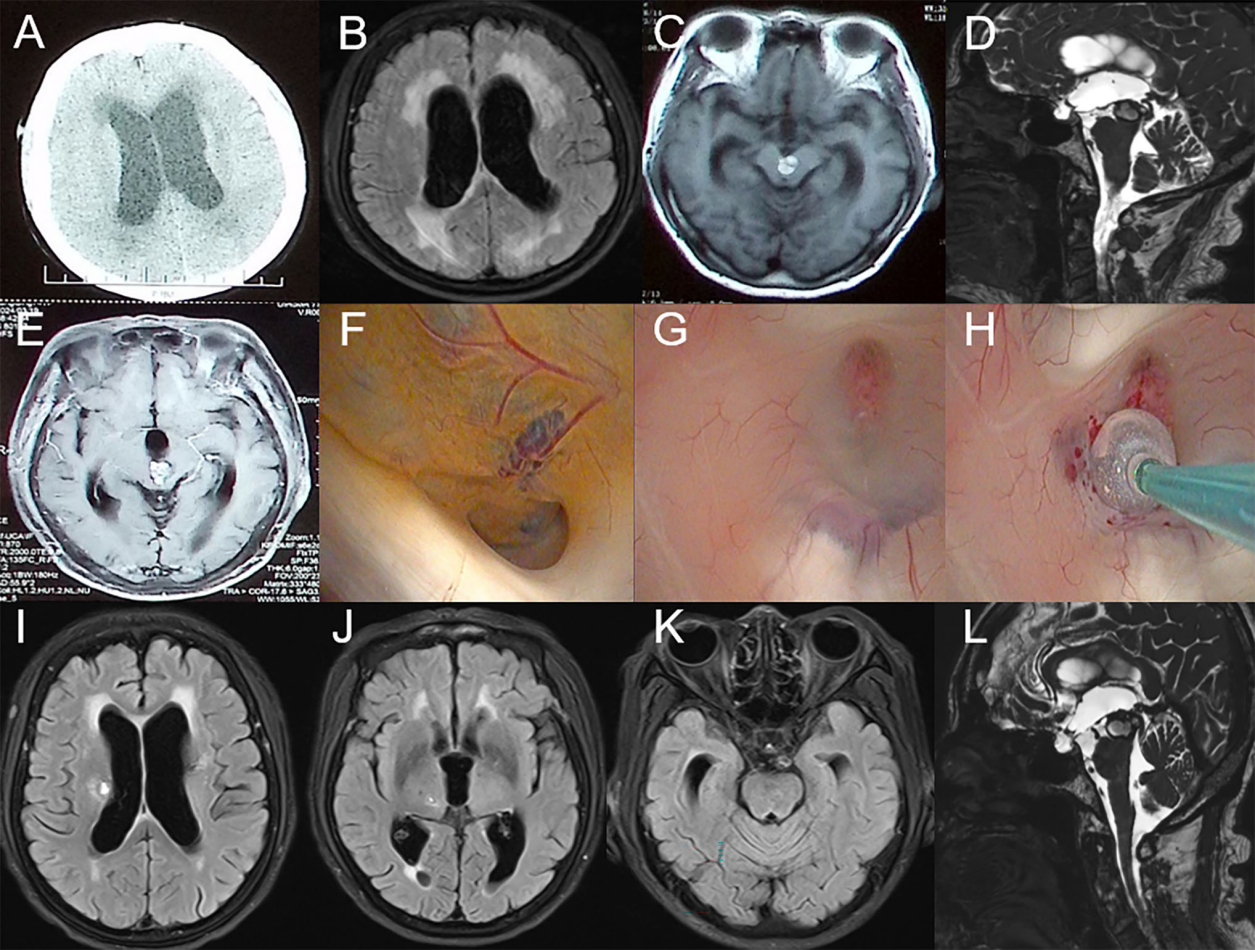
Preoperative imaging examination, intraoperative findings, and postoperative follow-up of the patient in Case 2. (A-E): CT and MRI reveal a mass in the midbrain and clear signs of obstructive hydrocephalus. (F): CCM obstructing the cerebral aqueduct with surrounding hemosiderin deposition. (G-H): Select the appropriate site and create the fistula. (I-L): MRI results on the seventh day postoperatively.
